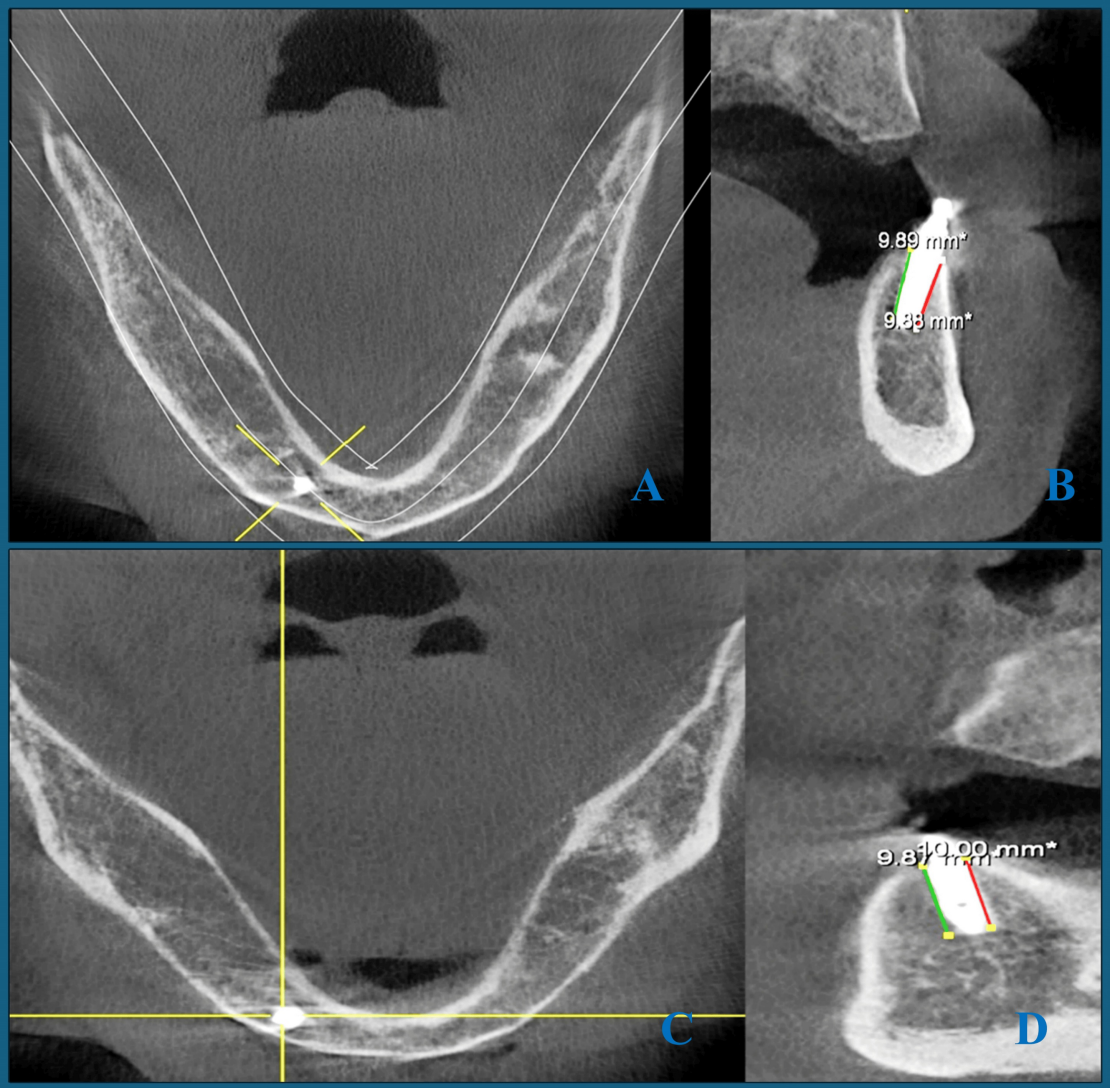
(A) Axial CBCT image displaying the panoramic reconstructed curve. (B) Reconstructed cross-sectional image displaying measurements of the buccal and lingual bone levels surrounding the implant. (C) Axial CBCT image displaying the alignment of the coronal section to pass through the implant. (D) Coronal image displaying measurements of the distal and mesial bone levels surrounding the implant CBCT: cone beam computed tomography
